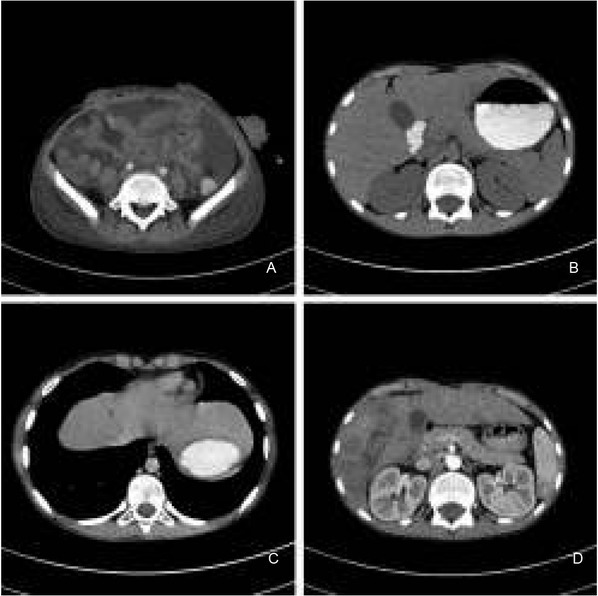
Further course of the disease as studied with computed tomography. A. Tomographic image of the abdomen after first surgery, large amounts of free fluid present. B. Abdominal tomography shortly after first surgery, no metastatic foci in the liver were detected. C. Abdominal tomography at four months after first surgery, note a small hypodense metastatic liver lesion. D. Computed tomography of the abdomen at a later time, numerous liver metastases. Ryc. 4. Dalszy przebieg choroby w obrazowaniu tomografią komputerową. A. Tomografia brzucha po pierwszej operacji - znaczna ilość wolnego płynu w jamie brzusznej. B. Tomografia brzucha bezpośrednio po operacji, z uwidocznieniem wątroby − bez dostrzegalnych ognisk przerzutów. C. Jama brzuszna w cztery miesiące po operacji - ognisko przerzutowe o niewielkiej hipodensyjności w środku wątroby. D. Jama brzuszna w późniejszym terminie - kolejne liczne przerzuty do wątroby.
